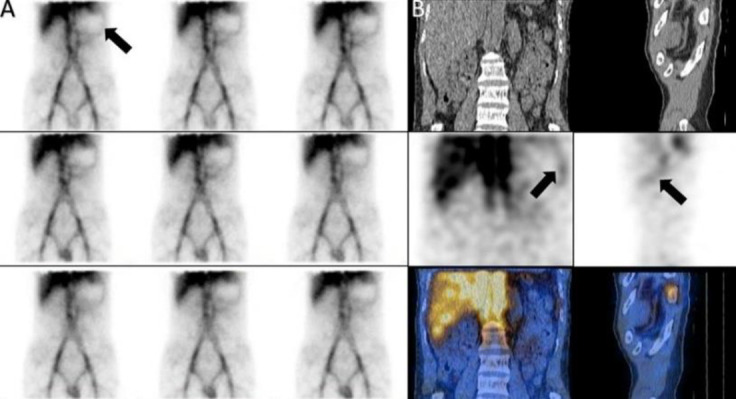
Right gastroepiploic vessels on planar (A) and SPECT/CT (B)
